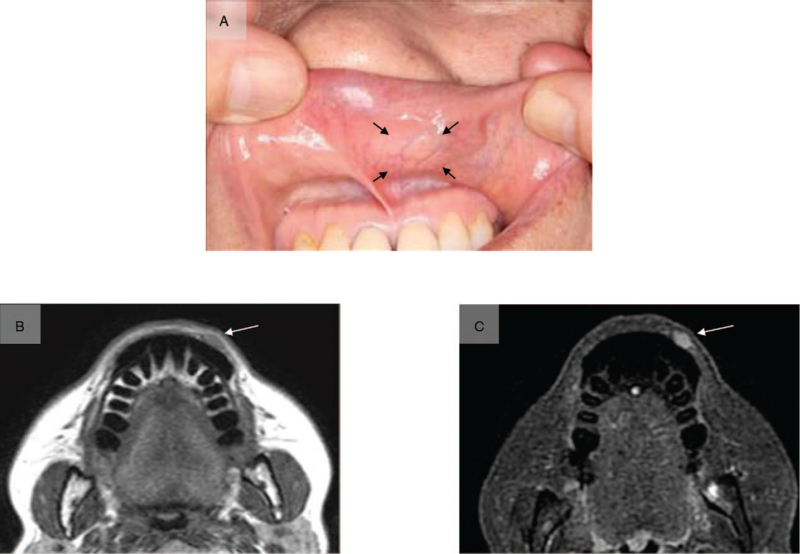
Clinical findings. An elastic-hard protruding mass measuring 15 x 10 mm was noted on the left side of the upper lip (A). The mass exhibited moderate intensity on contrast T1-weighted magnetic resonance imaging (B) and high intensity on short T1 inversion recovery (C). The continuity of the orbicularis oris muscle was retained.
